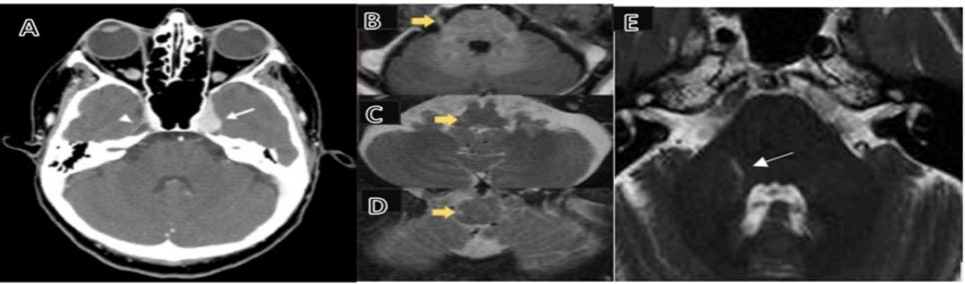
Imaging findings in trigeminal HZ and PHN. A Tumor-like enhancement (arrow) of the trigeminal ganglion, representing chronic VZV ganglionitis in a patient with ipsilateral PHN. B–D Axial T2 MRI hyperintensity of the right trigeminal nerve root, pons, and medulla in a patient with acute right V1 zoster (yellow arrows). E “Trigeminal pontine sign”—axial T2 MRI hyperintensity in a patient with right V1 trigeminal PHN (arrow). Reproduced from Refs. [38–40] (modified)
